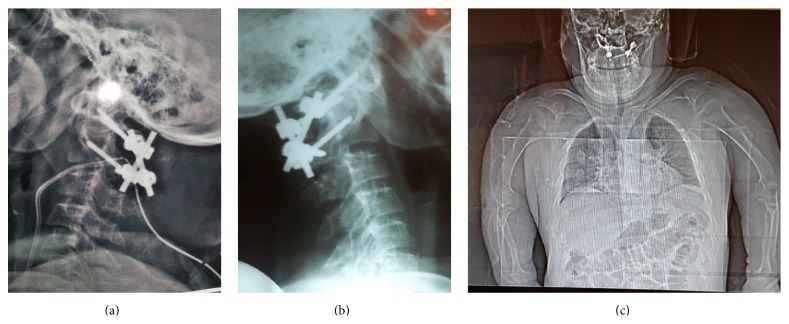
(a) and (b) Lateral cervical radiographs a day after surgery and a month after surgery. (c) AP radiographs showing this achondroplastic patient with instrumentation at C1-C2 region, note rhizomelic upper extremity and short statue of the patient.
